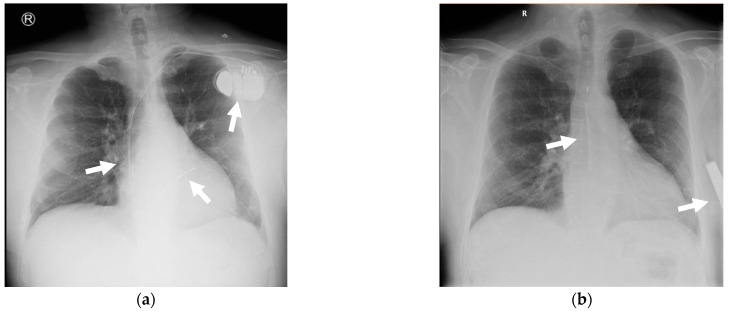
Chest X-ray: (a) on the left side (at admission), a bicameral ICD is visible, with arrows indicating the generator located in the left subclavicular region, the leads situated in the right atrium and right ventricle, respectively. On the right side (b) after S-ICD implantation, the chest X-ray shows arrows indicating the S-ICD electrode and the S-ICD generator.
